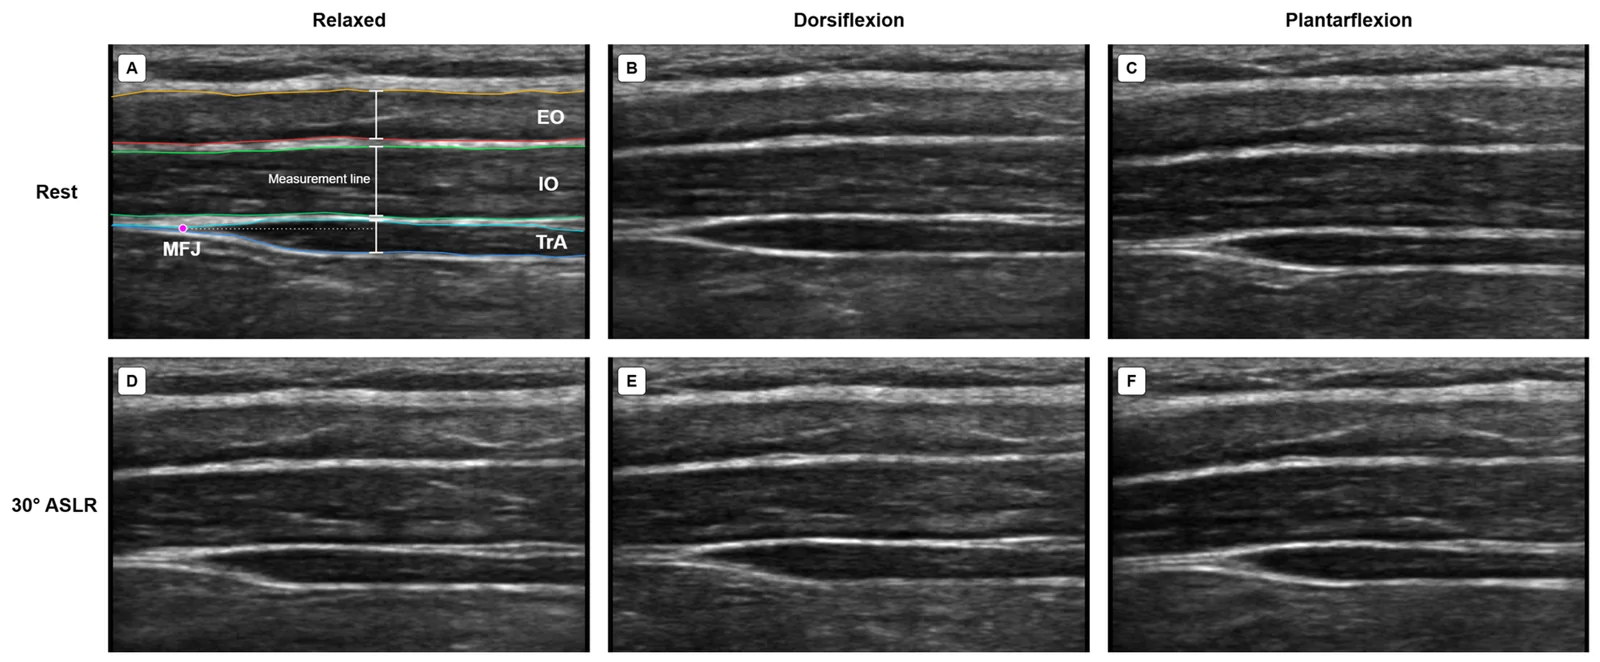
Representative B-mode ultrasound images of the lateral abdominal muscles at rest (top row) and during a 30° ASLR (bottom row) with a relaxed ankle, maximal active DF, or maximal active PF. Images were obtained from one participant using consistent settings and transducer placement. In panel (A), colored contours delineate the fascial borders of the EO, IO, and TrA; the magenta point marks the muscle fascia junction (MFJ), and the horizontal white dashed line indicates the 1.5 cm medial offset to the vertical measurement calipers. Panels (B–F) are unannotated to preserve anatomical visibility.
